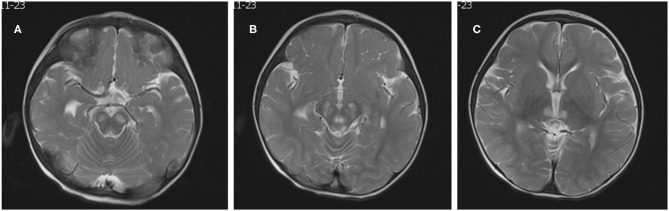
Neuroradiological features. Brain MRI showed bilateral, symmetric signal abnormalities in the basal ganglia, and brain stem (A–C: T2-weighted images).
